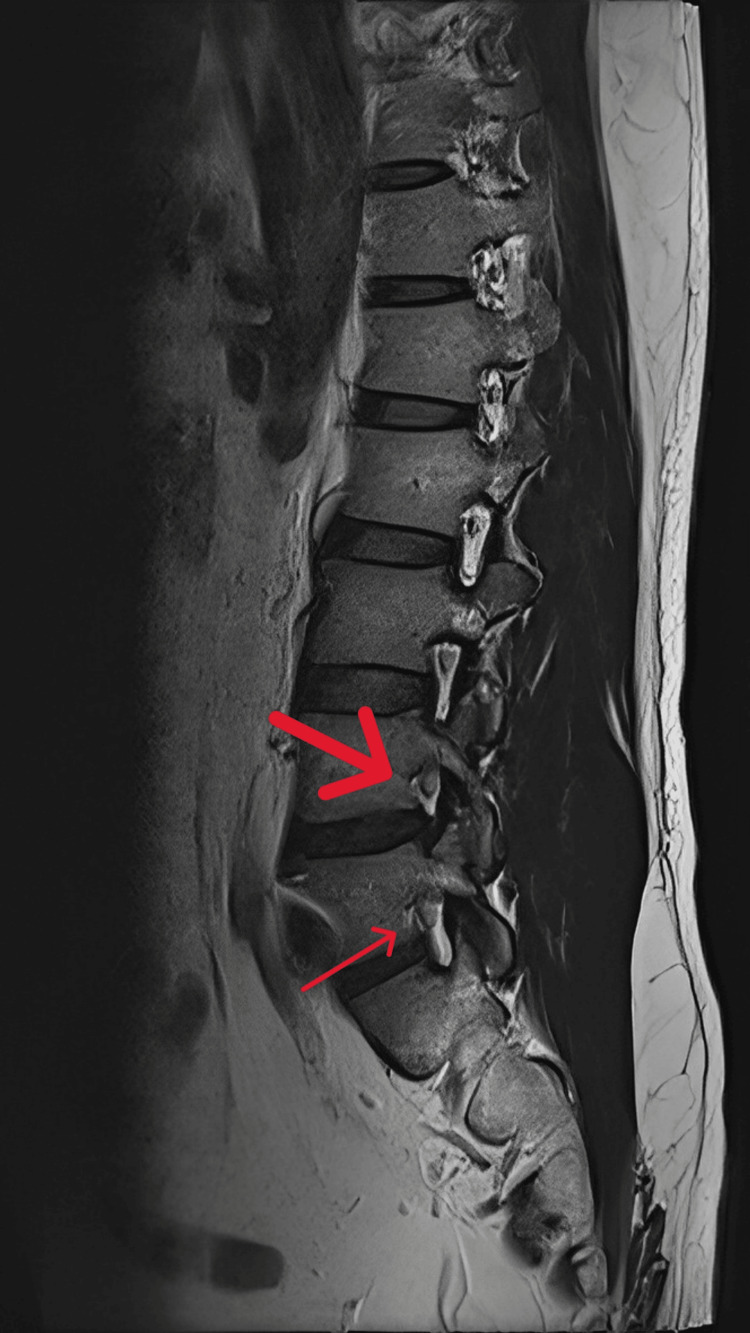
Parasagittal view of T1-weighted images of MRI, with a small red arrow indicating grade 1 stenosis, featuring compression of the epidural fat where the remaining fat still completely surrounds the nerve root, and a large red arrow indicating grade 2 stenosis, where the epidural fat only partly surrounds the nerve root
